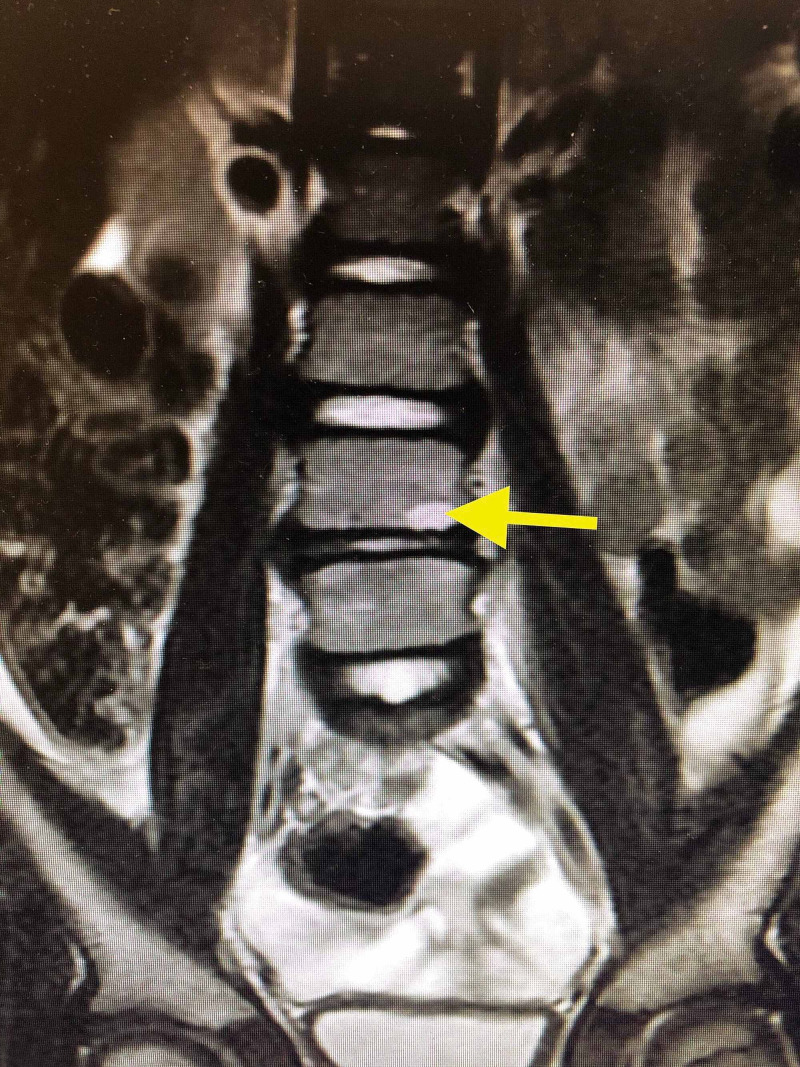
MRI scan showing vertebral erosive changes.
